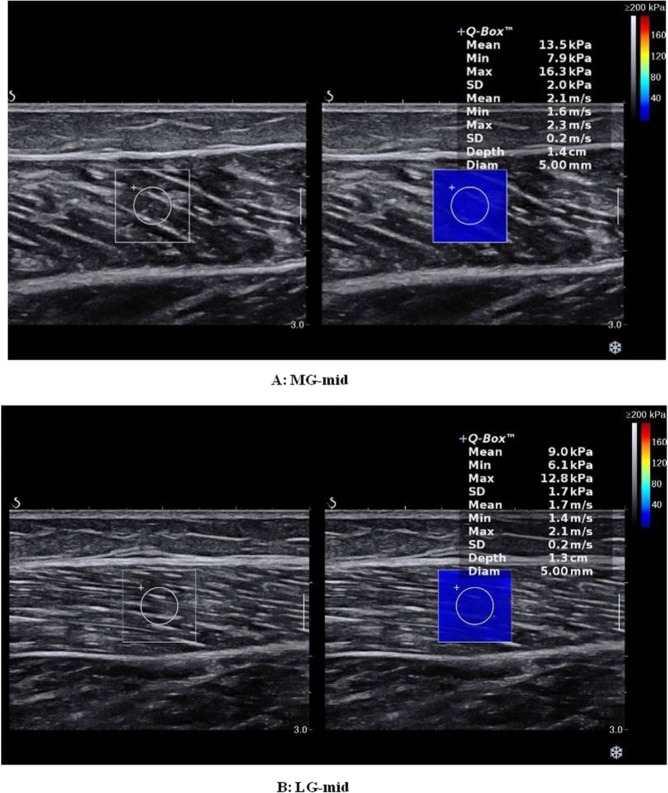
Longitudinal* shear wave elastography scale sonograms of the MG and LG show the measurement of the shear modulus. The color-coded box indicating muscle-tendon elasticity is shown in the upper images. The longitudinal grey-scale sonograms of muscle-tendon are shown in the bottom images. The Q-Box^TM^ is shown on the right. (A) Shear modulus values of the MG with the ankle joint in the relaxed position. (B) Shear modulus values of the LG with the ankle joint the relaxed position.
